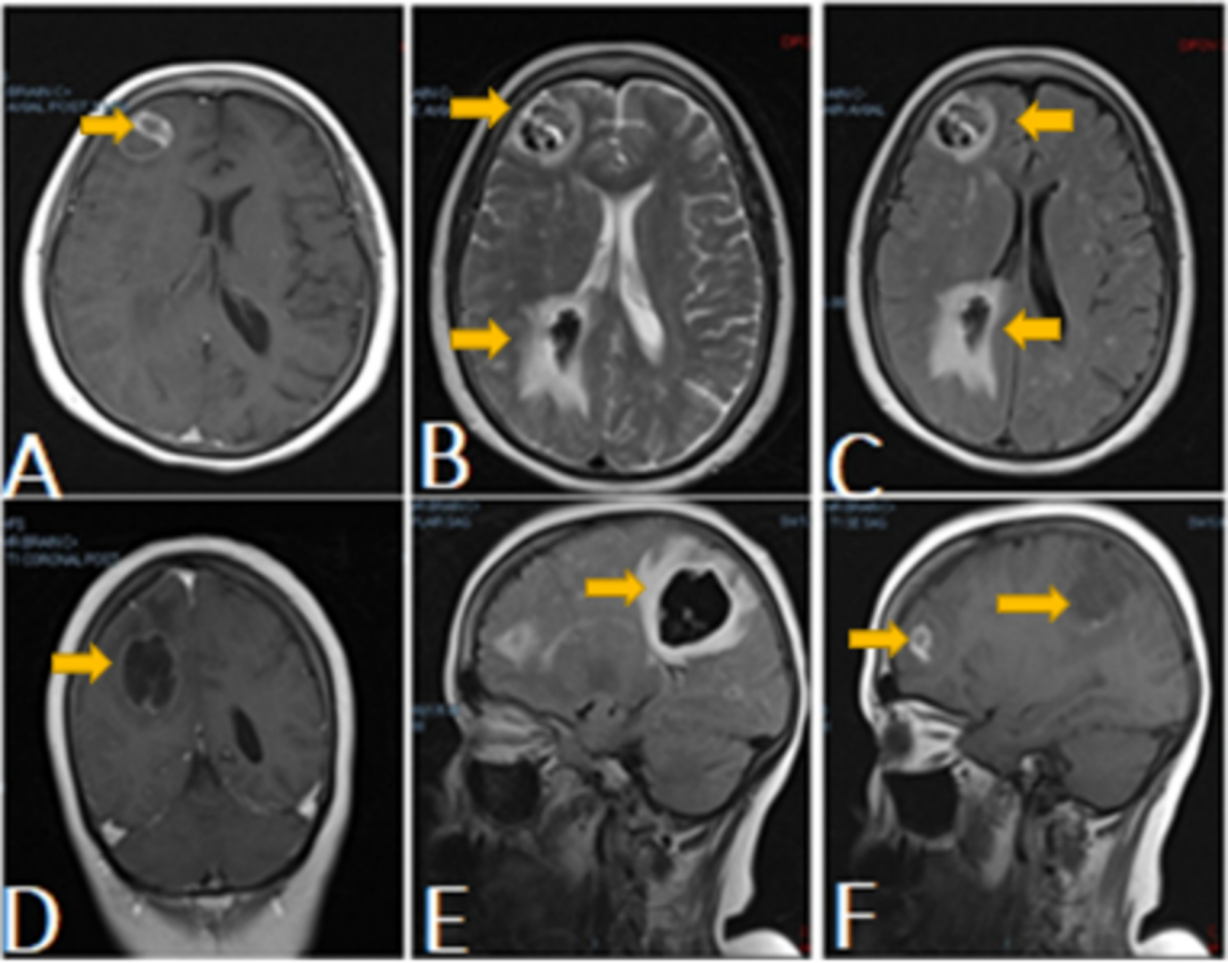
Magnetic resonance imaging (MRI) of the brain MRI of the brain (Figures A-C, axial view) revealed a peripherally enhancing right posterior parietal hematoma with central hypointensity and surrounding edema, along with a septated rim-enhancing focus about the right frontal lobe. Figure D represents the coronal view. Figures E-F (sagittal view) presented a redemonstration of right frontal and posterior parietal intraparenchymal hematomas.
